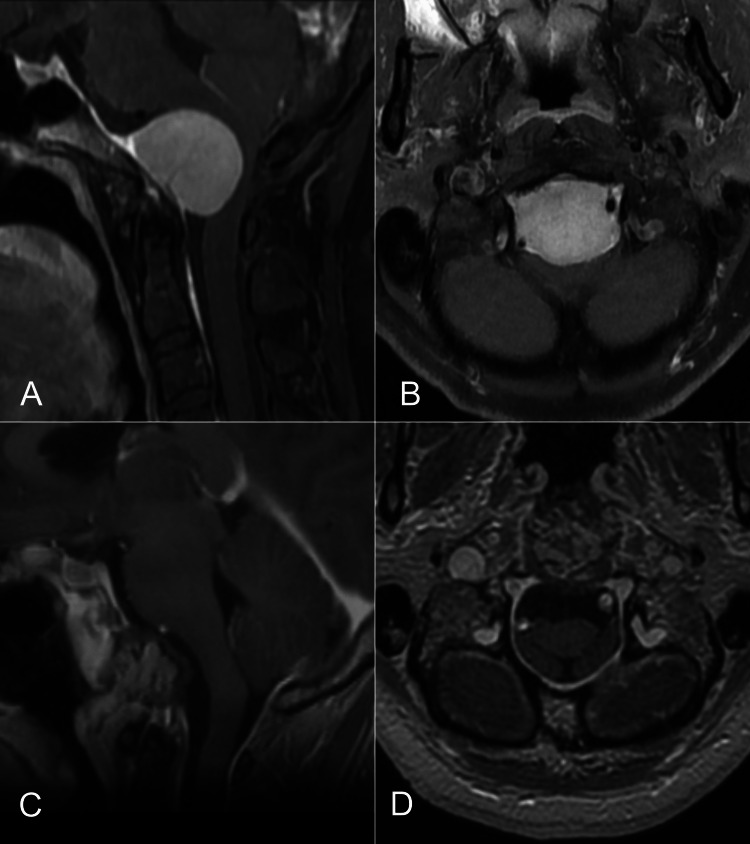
A representative case involves a 50-year-old female who presented with severe myelopathy and was diagnosed with a ventral foramen magnum meningioma. An endoscopic endonasal transclival approach was adopted, achieving Simpson grade I tumor resection. The patient experienced significant improvement in myelopathy symptoms postoperatively, with no new neurological deficits and no CSF leakage. She was able to return to her normal daily activities 2 weeks after the surgery. A, B Preoperative T1-weighted contrast-enhanced MRI: Sagittal (A) and axial (B) views demonstrate a large, ventral foramen magnum meningioma occupying the inferior clivus. The lesion causes significant posterior displacement of the brainstem and lateral displacement of the bilateral vertebral arteries. C, D Postoperative T1-weighted contrast-enhanced MRI: Sagittal (C) and axial (D) views 3 months after the surgery confirm total tumor removal and brainstem decompression. The reconstruction site shows a well-positioned vascularized flap and fat graft obliterating the bony defect
